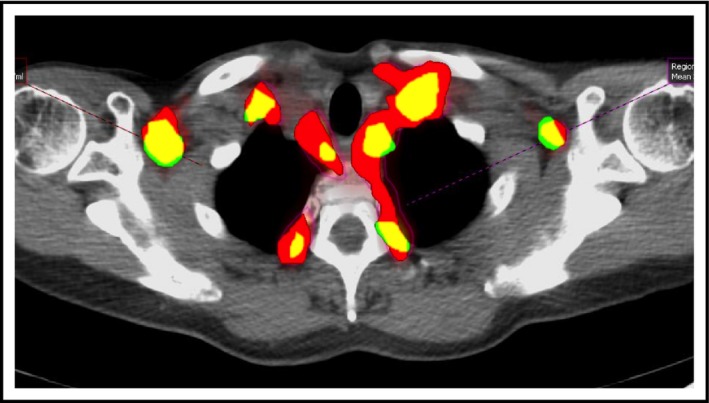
Axial PET/CT of the upper thorax for patient B showing superimposed segmented ^18^F‐FDG BAT regions of interest from the index (red) and subordinate PET/CT scans (green), with areas of colocalization shown as yellow (reprinted with permission of OmniScriptum GmbH (Jones 2016)).
